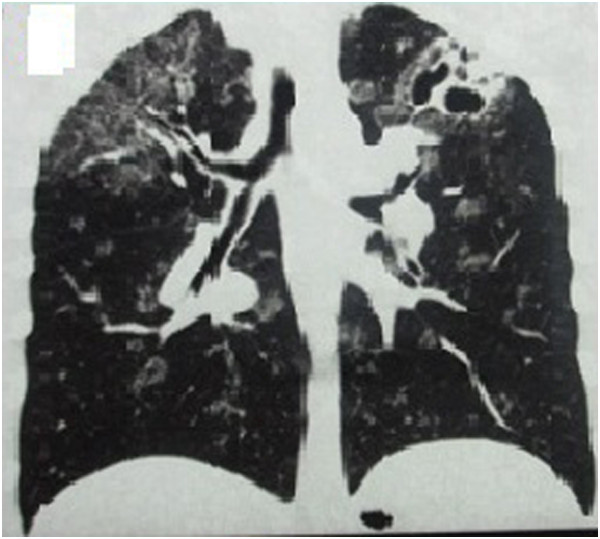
Chest HRCT showed that the lung lesions had worsened and that protrusions were present on the tracheal wall.
